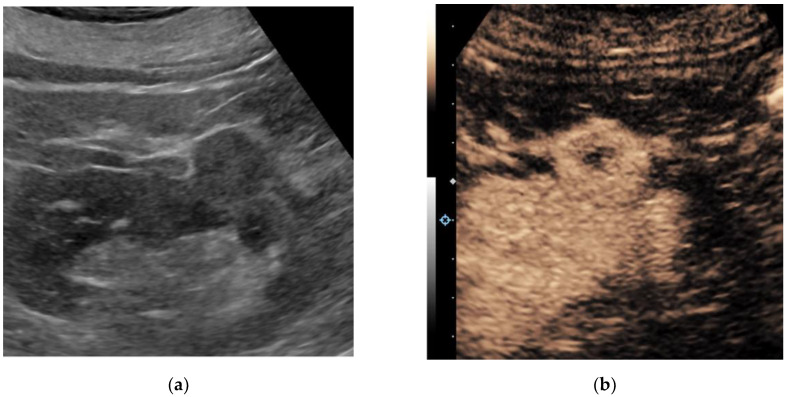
A 67-year-old man with multiple renal lesions, status post SBRT one year prior for contra-lateral RCC. Grayscale ultrasound image of the left kidney in longitudinal orientation (a) shows an isoechoic exophytic nodule. Following the intravenous administration of 1.0 cc Lumason ultrasound contrast, a contrast-enhanced ultrasound image (b) shows the nodule demonstrating predominantly solid avid enhancement relative to the adjacent renal cortex. A partial nephrectomy revealed clear cell renal cell carcinoma, grade 2.
